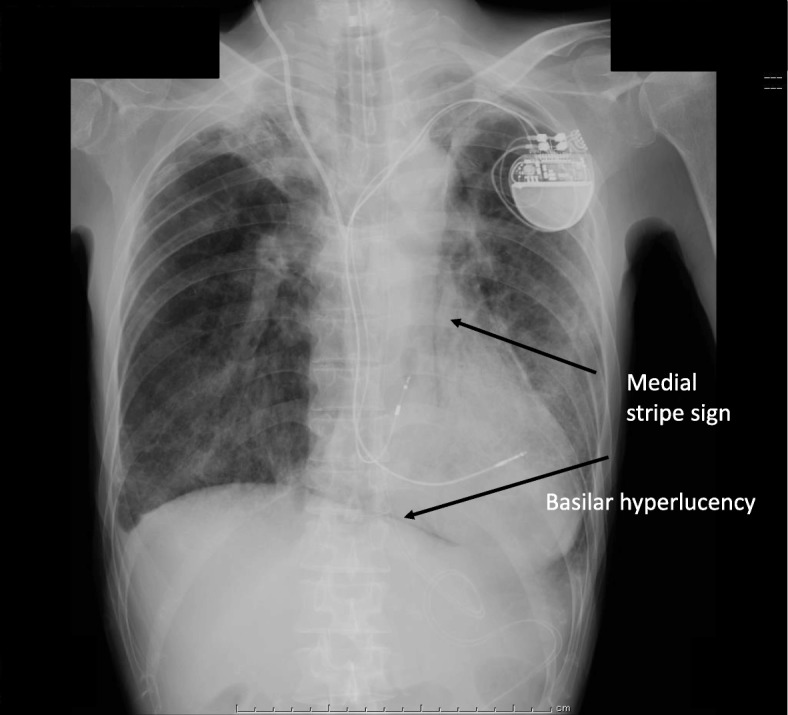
Pneumatosis. Chest X-ray shows a radiolucent outline around the heart and mediastinum. The arrows show a medial stripe sign and basilar hyperlucency
